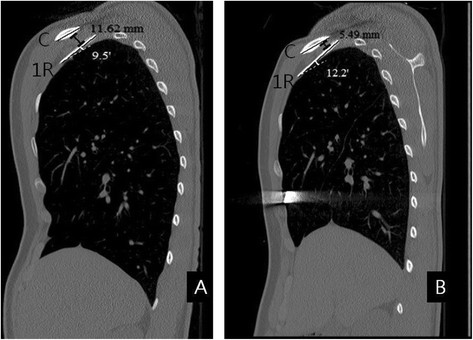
Postoperative changes of the costoclavicular space in patient with pectus excavatum. This patient was a 17- year- old male patient. The type of pectus excavatum was symmetric and the preoperative Haller index was 3.61. He underwent the Nuss procedure with a single metal bar. The shortest distance was defined as the shortest distance between the first rib and the clavicle on a sagital view of the 3-dimensional chest CT (black color). When the distance between the first rib and the clavicle was shortest, the angle between the long axis of the first rib and the clavicle was defined as the crossing angle (white color). Although the postoperative arm positions were more adducted than the preoperative arm positions bilaterally, the shortest distance became decreased and the crossing angle became increased, postoperatively (Figure A: preoperative status. Figure B: postoperative status, C: the clavicle, 1R: the first rib).
